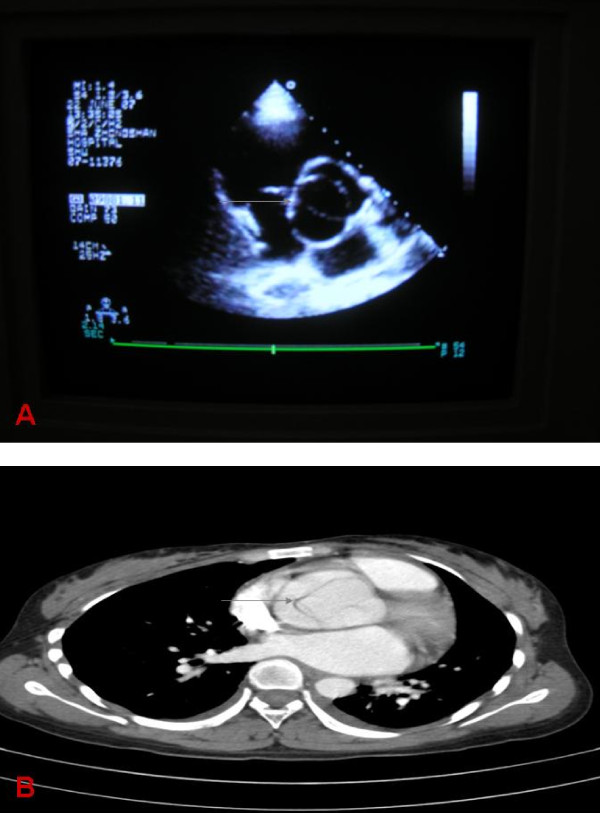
Aortic dissection in case II with bicuspid aortic valve. (A) Echocardiography: Bicuspid aortic valve (grey arrow). (B) CT scan: Ascending aortic dissection (grey arrow).
